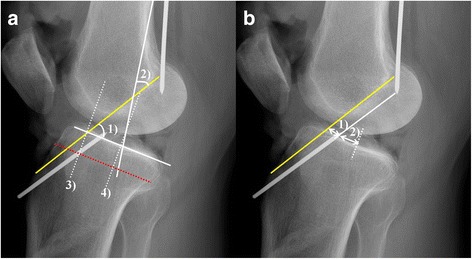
Measurement of radiographic landmarks in full extension lateral radiographs. a 1) Angle between Blumensaat’s line and the tibial articular surface, 2) Angle between Blumensaat’s line and the posterior femoral cortex, 3) Sagittal location of Blumensaat’s line in Amis & Jakob’s line, 4) Sagittal location of the apex of the tibial eminence in Amis & Jakob’s line. b 1) Distance from Blumensaat’s line to the center of the ACL tibial footprint, 2) Distance from the apex of the tibial eminence to the center of the ACL tibial footprint
